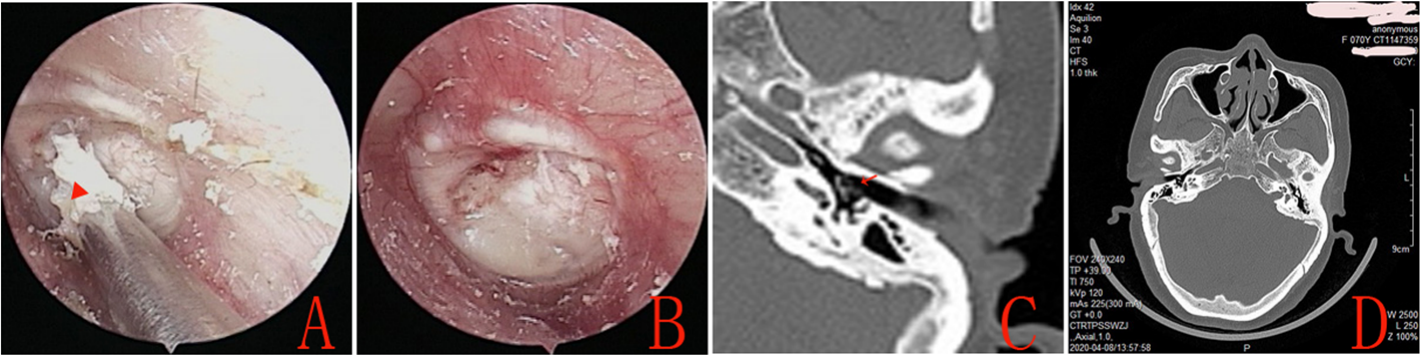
The removal of epithelial pearls (Red triangle) under endoscope (a), the eardrum following the removal of epithelial pearls (b), CT revealed the eardrum (Red arrow) and well pneumatized middle ear (c and d). Please note, this is the same patient as in Fig. 4
